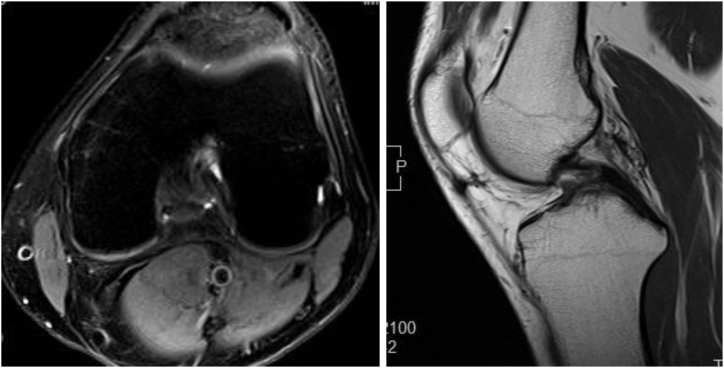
One-year postoperative magnetic resonance imaging of the left knee revealing no evidence of recurrence, indicating the efficacy of the arthroscopic technique in achieving complete resection.
